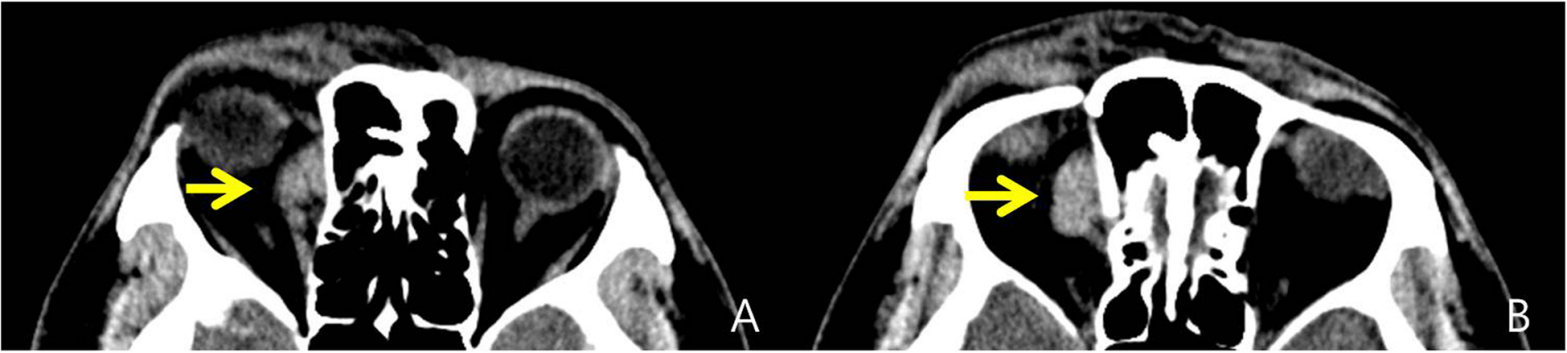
Axial computed tomography scans show a homogenous well-enhanced mass (2.4 × 1.5 cm) at the superomedial aspect of the right orbit
